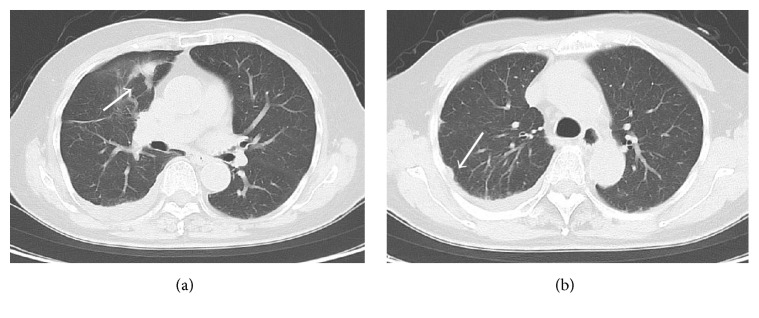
CT images showed nodular lesions in the right lung (a, b →).
